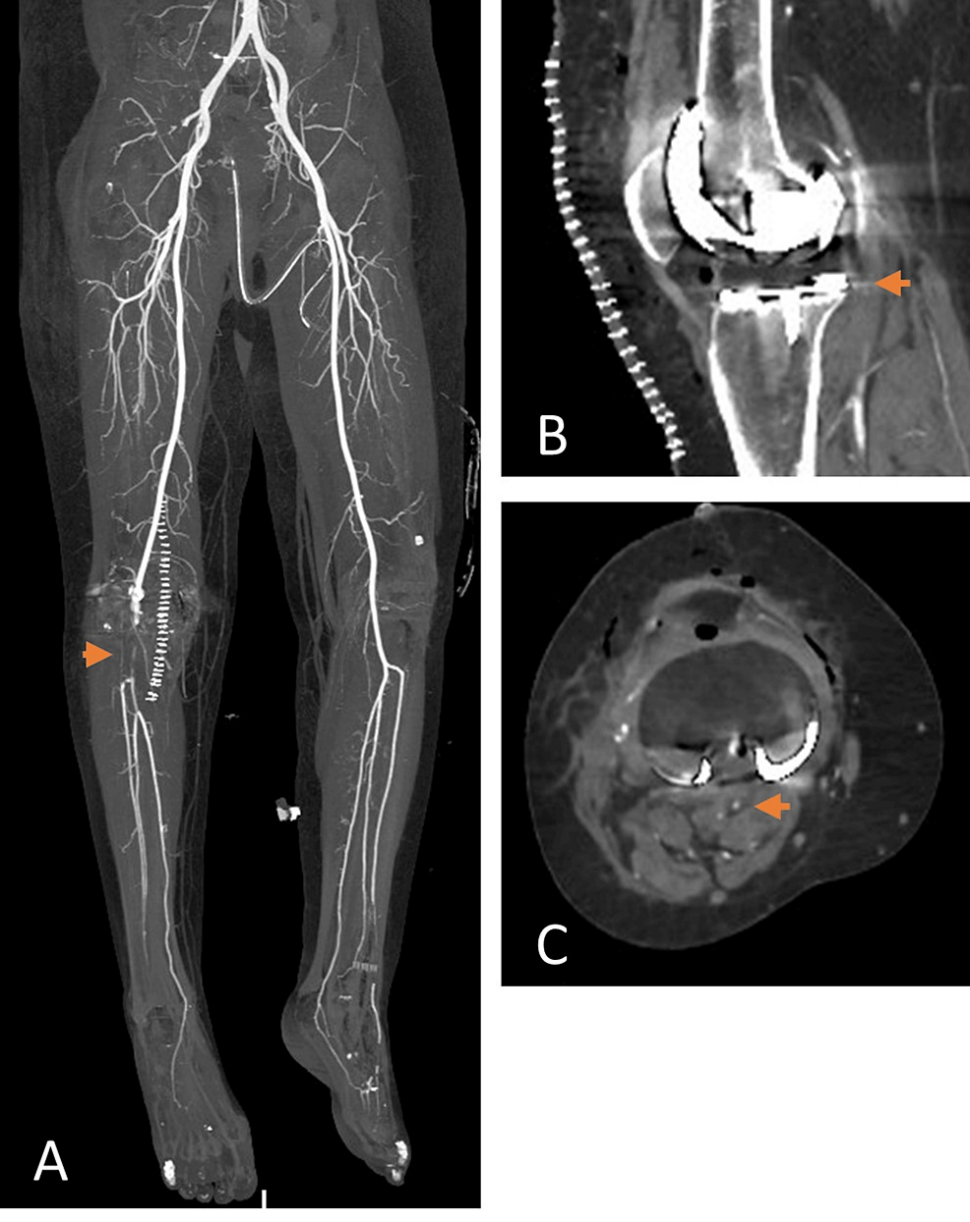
(A-C) Coronal, sagittal and axial images of computed tomography angiogram showing 6 cm thrombotic occlusion of the right popliteal artery extending to TP trunk. TP: Tibioperoneal
